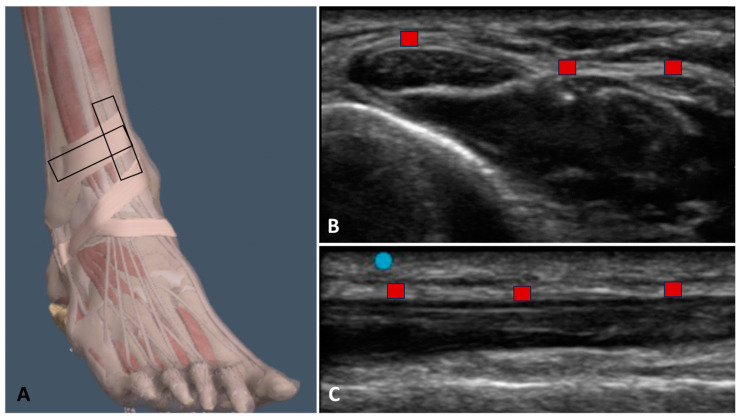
Ultrasound measurement protocol of SEAR thickness. (A) Starting from the transversal axis to the longitudinal axis, rotating by 90° degrees and sliding medially to laterally to acquire three points of evaluation on the longitudinal axis. (B) Transversal axis. (C) Longitudinal axis.
